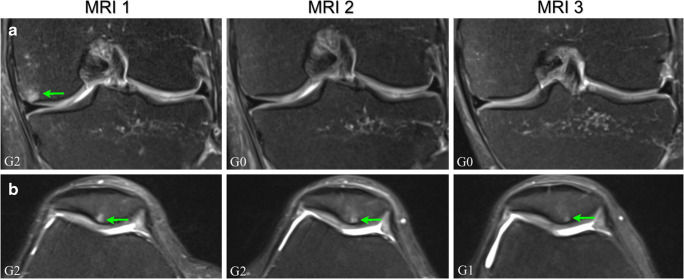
Coronal and axial proton-density fat-saturated MR images of two different knees with changes in the extent of subchondral bone marrow oedema-like signal: A) sustained improvement at 6-month follow-up (MRI 3) of a previous pre-marathon lesion (MRI 1) that reduced in extent 2 weeks after the marathon (MRI 2), in the femur of the left knee of a 54-year-old man; B) new improvement at MRI 3 in a pre-marathon lesion that remained unchanged from MRI 1 to MRI 2, in the patella of the right knee of a 48-year-old woman. Bone marrow oedema-like signal is indicated by arrows and the lesion grade (G) is included in the left bottom corner and is defined in the KOSS scoring system [18] (see Table 2); KOSS, Knee Osteoarthritis Scoring System
